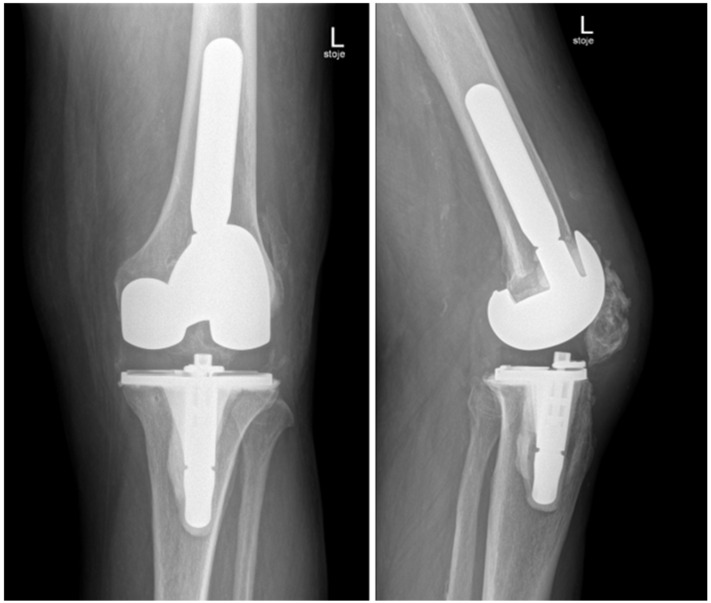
Radiography of the left knee—anteroposterior and lateral views after reimplantation of revision knee endoprosthesis.
